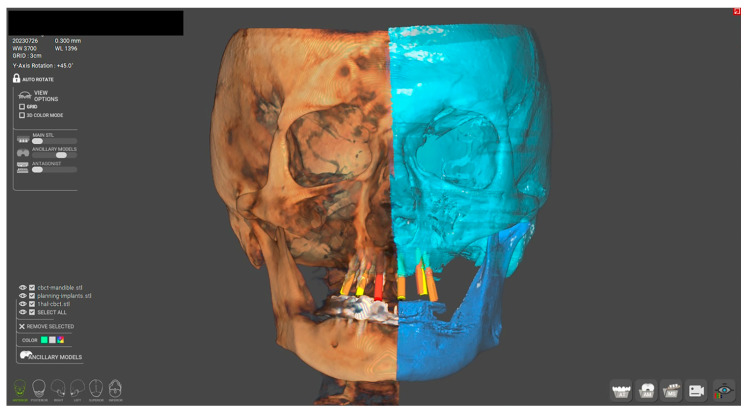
Aligned preoperative CBCT with treatment plan (right) and postoperative CBCT (left) in R2Gate software.
